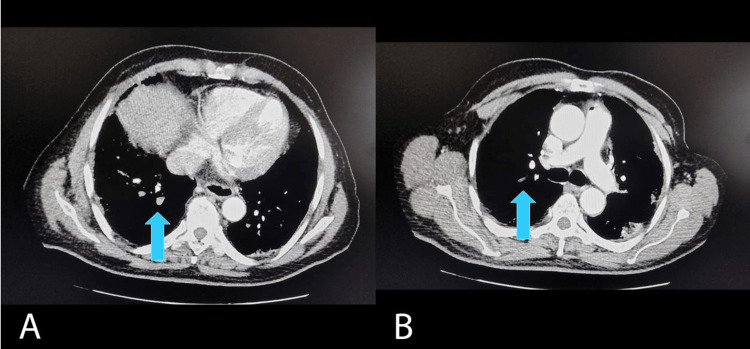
Images A and B show angio-CT scans with segmental and subsegmental pulmonary embolisms due to COVID-19, respectively
